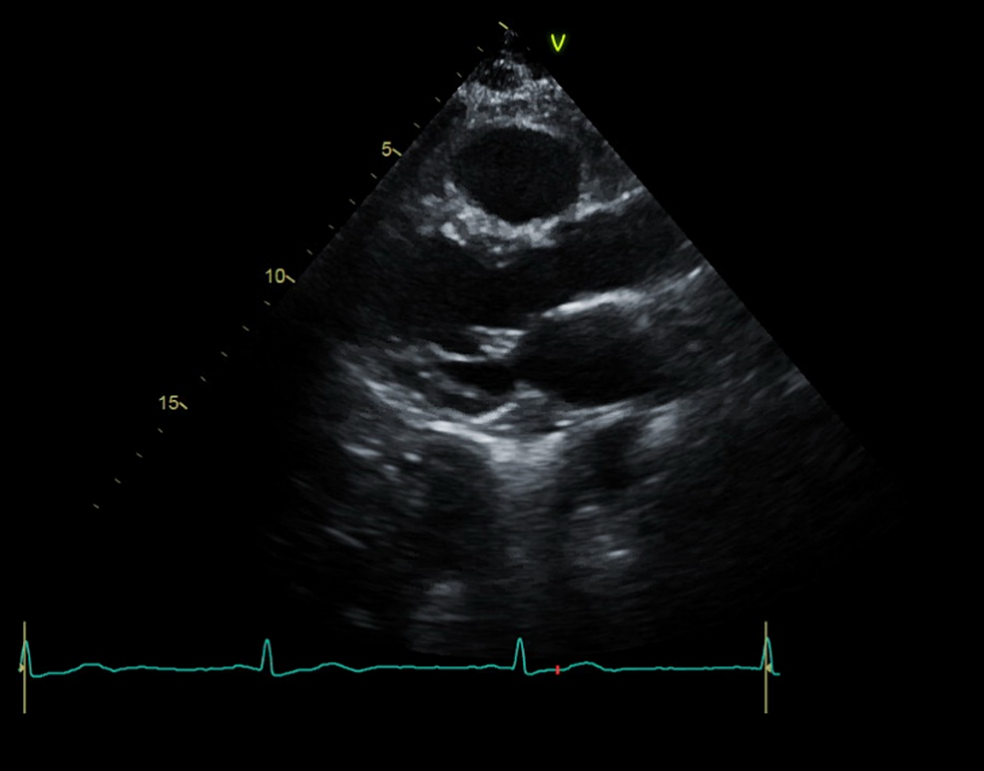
Parasternal long axis view of transthoracic echocardiogram unrevealing for structural abnormalities.
